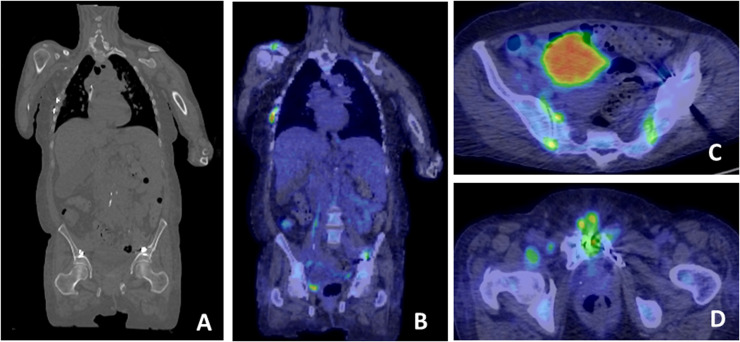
^18^F-FDG-PET/CT used to reveal occult foci of infection ^18^F-FDG-PET/CT was used to look for occult foci of infection in a case of recurrent *S. aureus bacteraemia* (A) This shows permanent implanted metal work in the right ribs and in the pelvis (B) This reveals possible foci of FDG avid infection at the right acromioclavicular joint and right ribs (C) FDG-avid bilateral sacroiliitis D: FDG-avid osteomyelitis of the pubic symphysis associated with metalwork. (Color version of figure is available online.)
